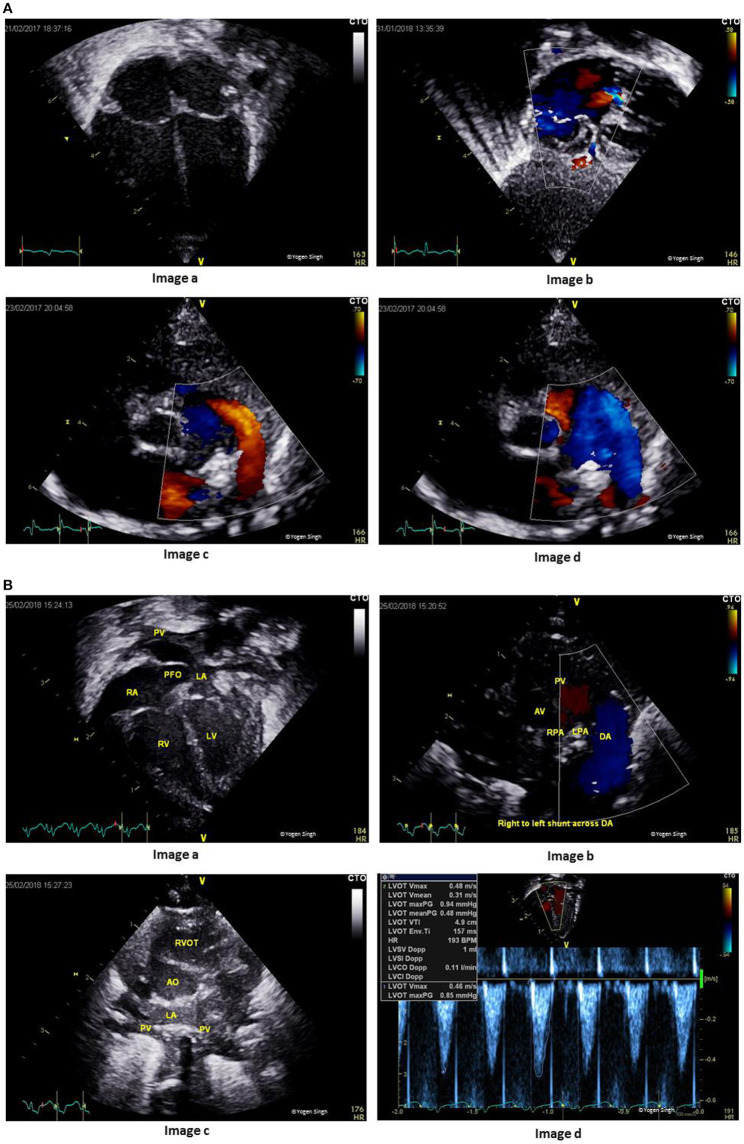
(A) Echocardiographic assessment during transitional circulation. Image “a” shows mild right ventricular dominance (physiological); Image “b” shows bidirectional shunt across foramen ovale; Image “c” shows left to right shunt across ductus arteriosus; and Image “d” shows right to left shunt across ductus arteriosus. Image “c” and image “d” are still frames from bidirectional shunt during early transitional circulation (which may be normal as compared to adverse adaptation in Figure 1C). (B) Echocardiographic assessment during adverse adaptation of transitional circulation in a preterm infant with sepsis at 8 h of age. Image “a” shows dilatation of right side of the heart in apical 4-chamber view with inter-atrial septum bowed toward left atrium (LA); Image “b” shows pure right to left shunt across ductus arteriosus (DA) because of high pulmonary vascular resistance (PVR) and low systemic vascular resistance (SVR); Image “c” shows small LA from decreased pulmonary return (decreased pulmonary blood flow due to high PVR and right to left shunt across DA; and Image “d” shows decreased left ventricle cardiac output (from decreased LV preload and impaired LV function).
